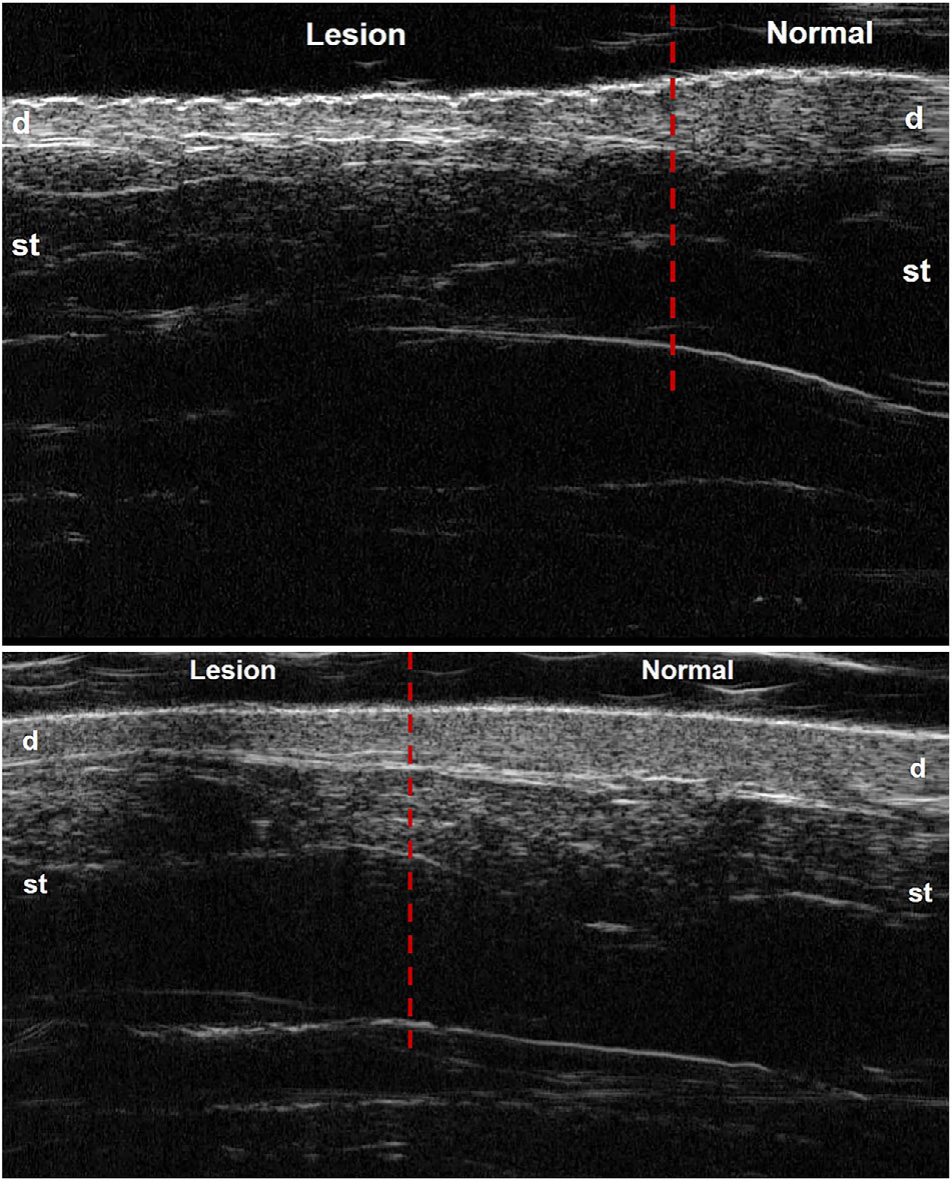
Ultrasonic images (Frequency: 50 MHz): a. The dermal and subcutaneous thickness decreased in Case 1; b. The decreased dermal thickness and normal subcutaneous tissue in Case 2. (d, derma; st, subcutaneous tissue)
